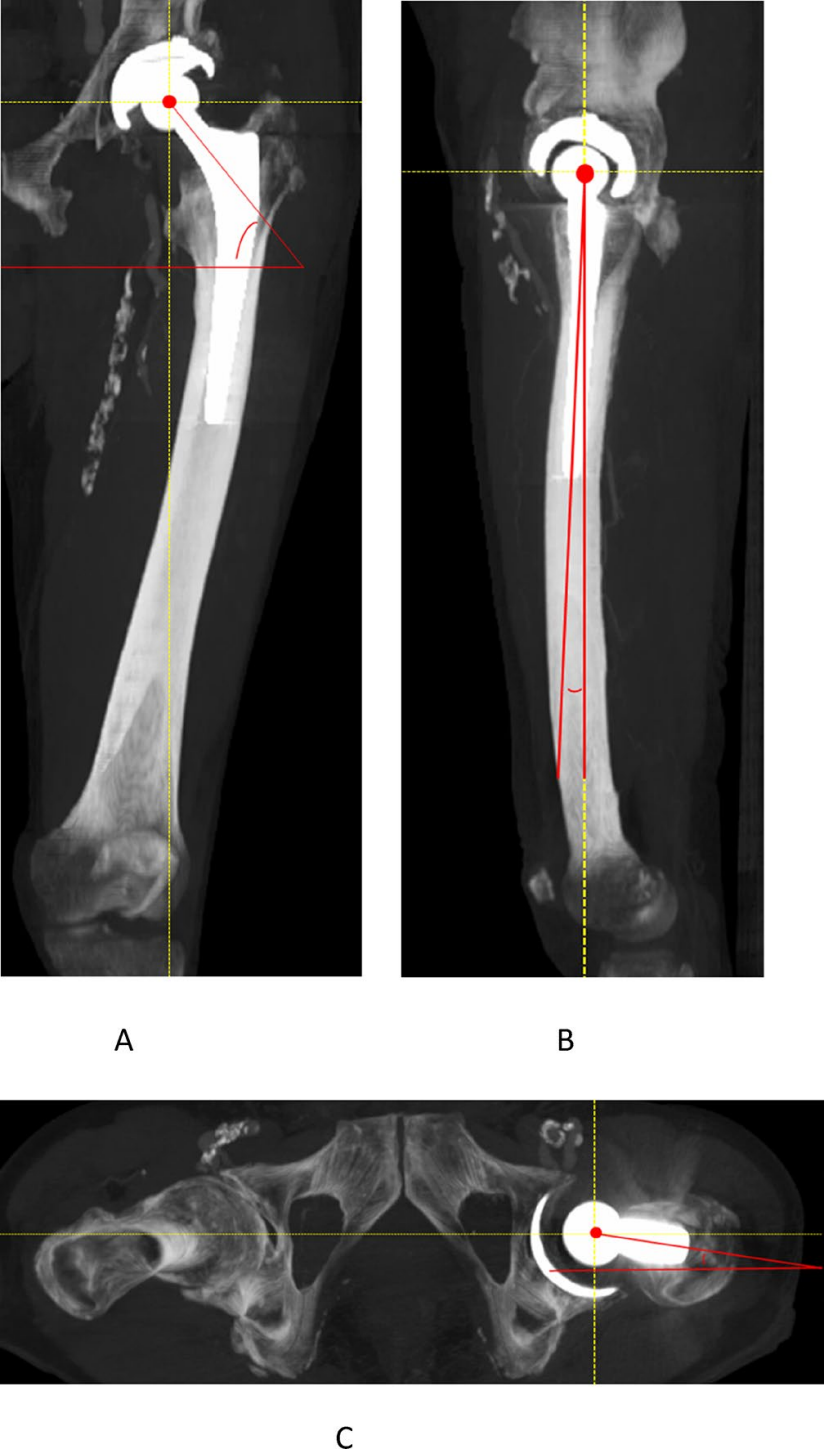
Measurement method of coronal inclination (CI_F_, defined as the angle between the line through the longitudinal axis of the neck of the femoral component and the horizontal) (A), sagittal inclination (SI_F_, defined as the angle between the line from the center of the femoral head to through the middle of the femoral neck, in relation to the vertical) (B) and transverse version (TV_F_, defined as the angle from the center of the femoral head through the femoral neck, in relation to the horizontal (C) of the femoral component on 3-D MIP constructed CT (horizontal/vertical displayed as dashed line).
